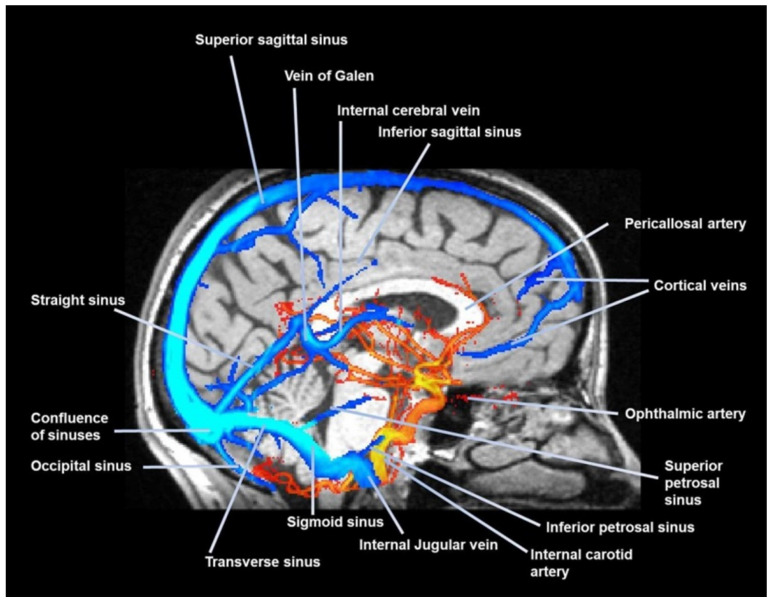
A sagittal view with magnetic resonance imaging. The image shows the venous sinuses and some arteries of the skull in a healthy subject. The photo was taken by Bruno Bordoni.
